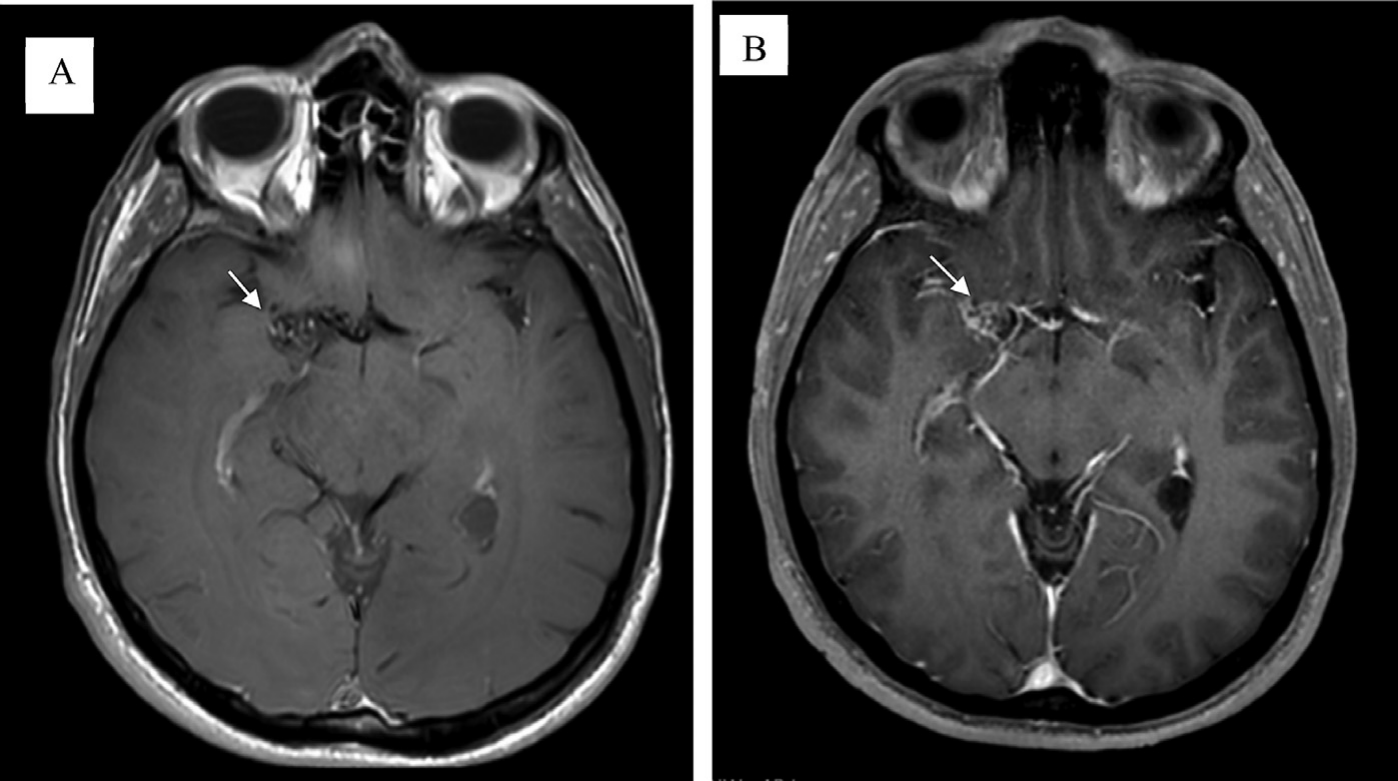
Magnetic resonance (MR) image. MRI Axial T1 post contrast in right MCA shows increased number and size of lenticulostriate vessels “puff of smoke” (images A and B) (white arrows) that represent collateral circulation as a consequence of tapering in right middle cerebral artery.
